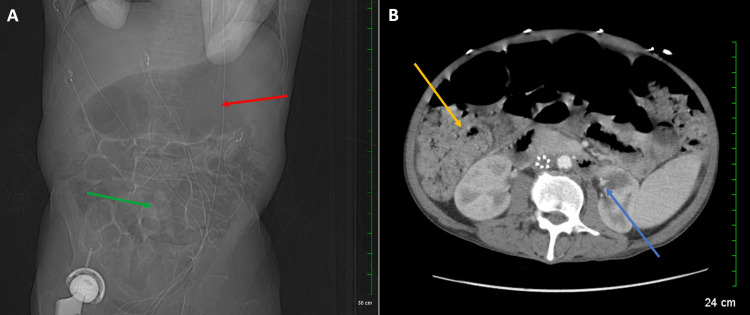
CT scan of the abdomen and pelvis with IV contrast (A) The abdomen showing an air-filled markedly distended stomach (red arrow) and a distended small bowel (green arrow). (B) The pelvis showing dilated loops of the bowel with a large amount of retained stool in the colon (yellow arrow) and a vague low-density region identified in the upper pole of the left kidney (blue arrow). CT: computed tomography, IV: intravenous
